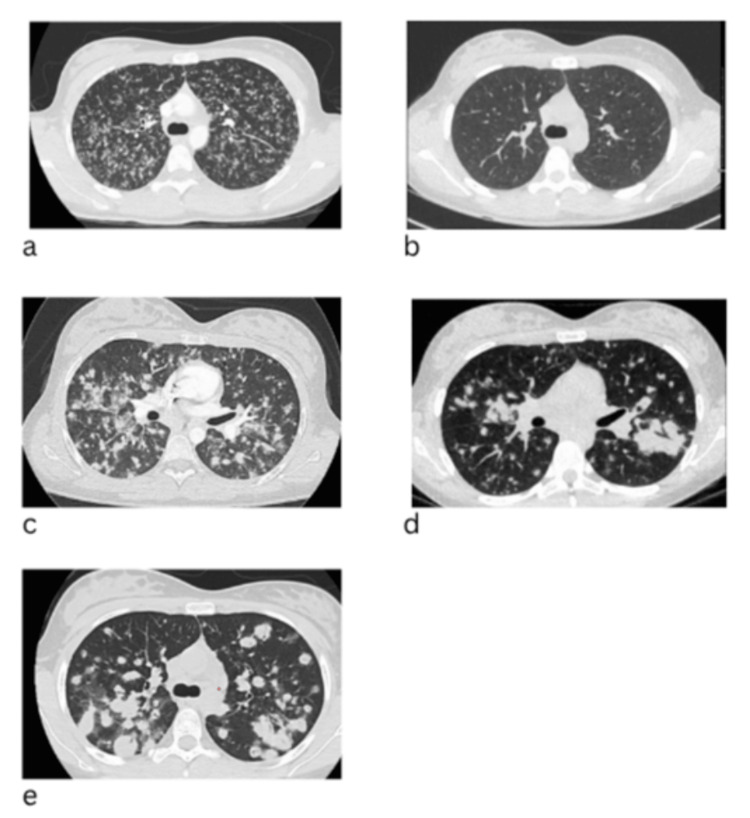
CT scan progression Image a: Reveals diffuse ground-glass and solid miliary nodules bilaterally with mild hilar adenopathy. Image b: Marked steroid response; Showed near complete resolution of the miliary micro nodularity, with only a few residual nodules. Image c: Six-month CT revealed recurrent diffuse miliary nodules that had increased in size (5-10 mm) and become more solid. Image d: CT chest three months after showed persistent, enlarging nodules. Image e: Chest CT demonstrating diffuse bilateral nodules with areas of coalescence and a 5.6-cm peripheral cyst/bulla in the right upper lobe.
